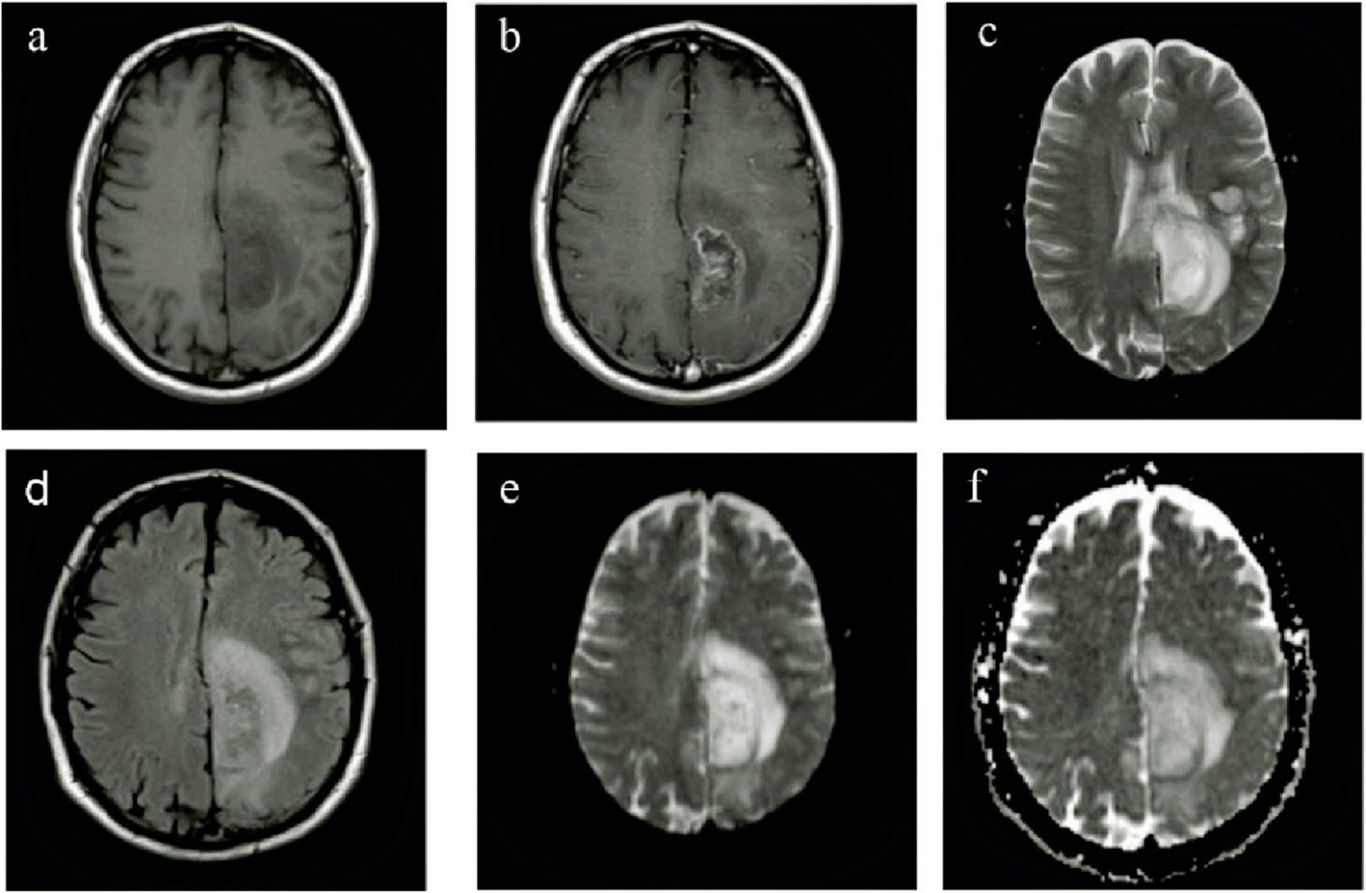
Axial views of a patient with ependymoma at the time of pre-operation: a T1-weighted sequences b T1-weighted sequences + Gd c T2-weighted sequences d Fluid-attenuated inversion recovery (FLAIR) sequences e Trace, diffusion-weighted imaging (DWI) f Apparent diffusion coefficient (ADCmap)
